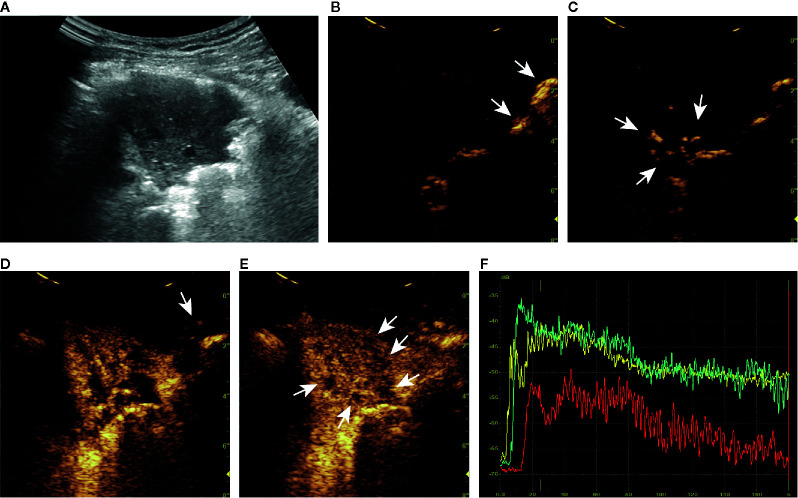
A series of ultrasound images of a benign subpleural pulmonary lesion (tuberculosis) in the upper lobe of left lung of a 61-year-old woman. B-mode ultrasound (A) showed the largest section of the lesion, and the angle between lesion border and thoracic wall was acute. The ultrasound contrast agent arrived at air-filled lung tissues (arrow) at about 4 s (B), the lesion (arrow) at 7 s (C) and the thoracic wall (arrow) at 14 s (D). At about 15 s, the lesion was completely enhanced and the intensity reached the peak (E). There was a tree-like vascular sign (D) and sieve-like non-enhancing regions (arrow) in the lesion (E). Accurate quantitative contrast-enhanced ultrasound parameters were obtained from the time-intensity curves of air-filled lung tissues (yellow curve), the lesion (green curve), and the thoracic wall (red curve) (F): basic intensity = -67.64 dB, lung-lesion arrival time difference = 2.56 s, ratio of arrival time difference = 26.18%. The malignant probability calculated by the ultrasound prediction model was 0.55% < 45.59%, so the lesion was predicted to be benign, which was consistent with the definite diagnosis. The corresponding dynamic clip is shown in Supplementary Video 1.
